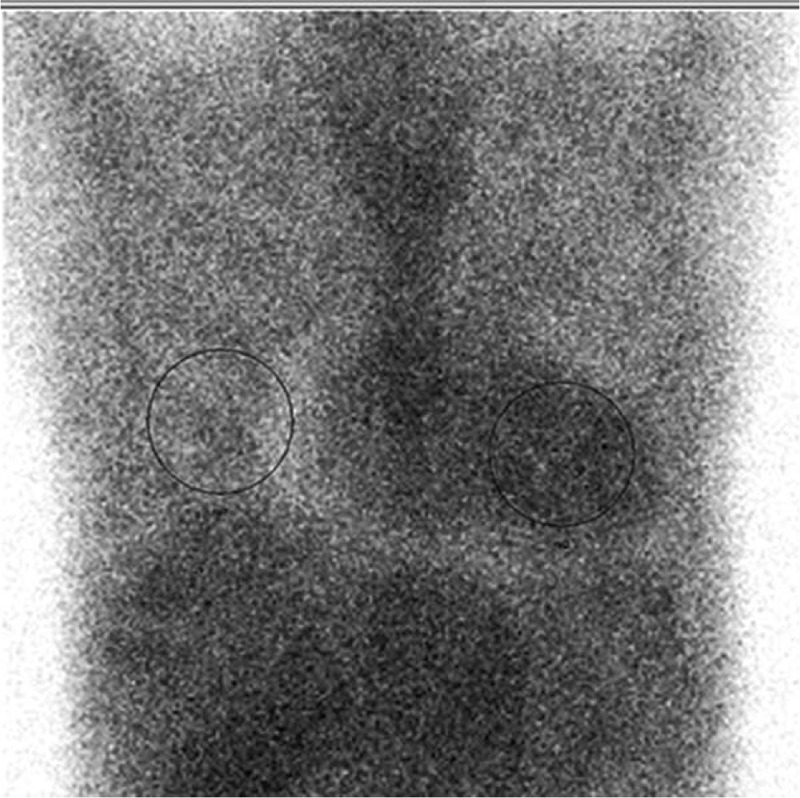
Tc-99m PYP scintigraphy. Intensive Tc-99m PYP uptake on nuclear scintigraphy. Tc-99m PYP scintigraphy revealed an intensive cardiac tracer uptake. The heart radio activity is equal to that of the ribs (grade 2).
